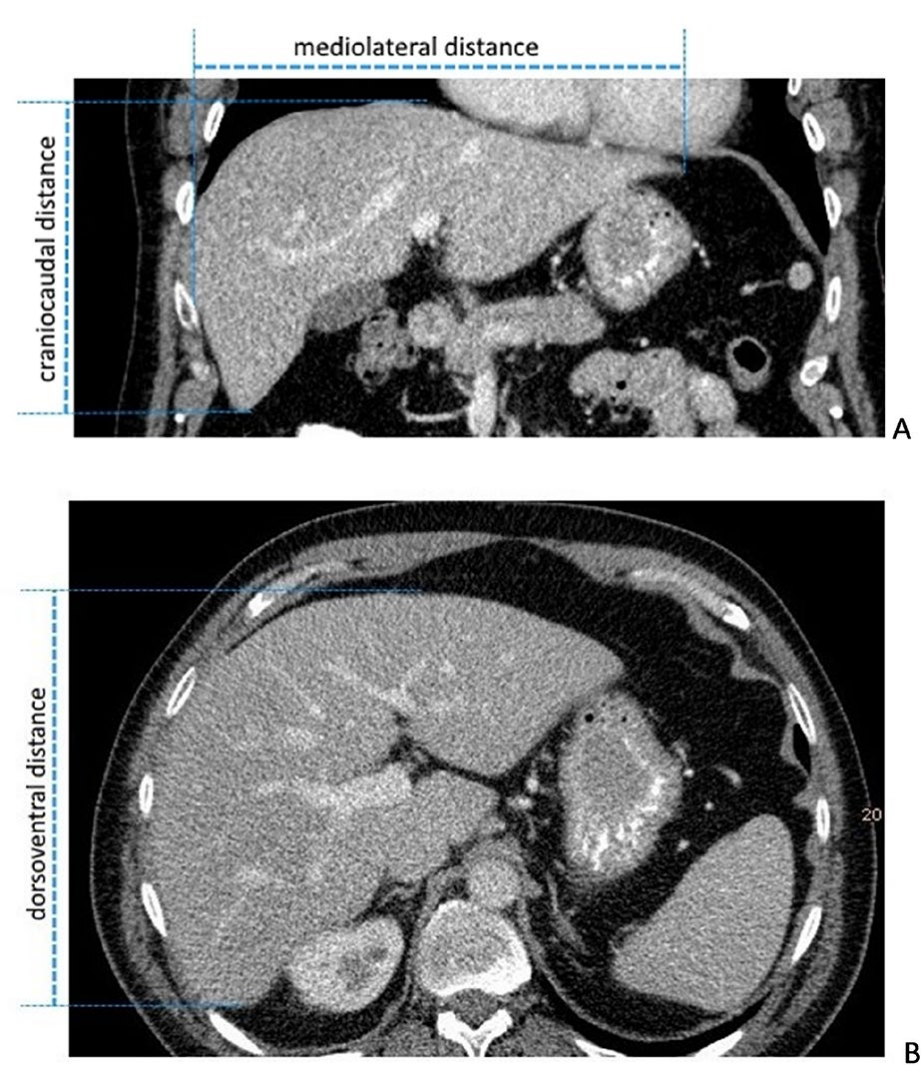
Representative measurement of maximum diameters in CT. Measurements were taken in the three standard dimensions (A) mediolateral (CTmaxML) + craniocaudal (CTmaxCC) and (B) dorsoventral (CTmaxDV).
